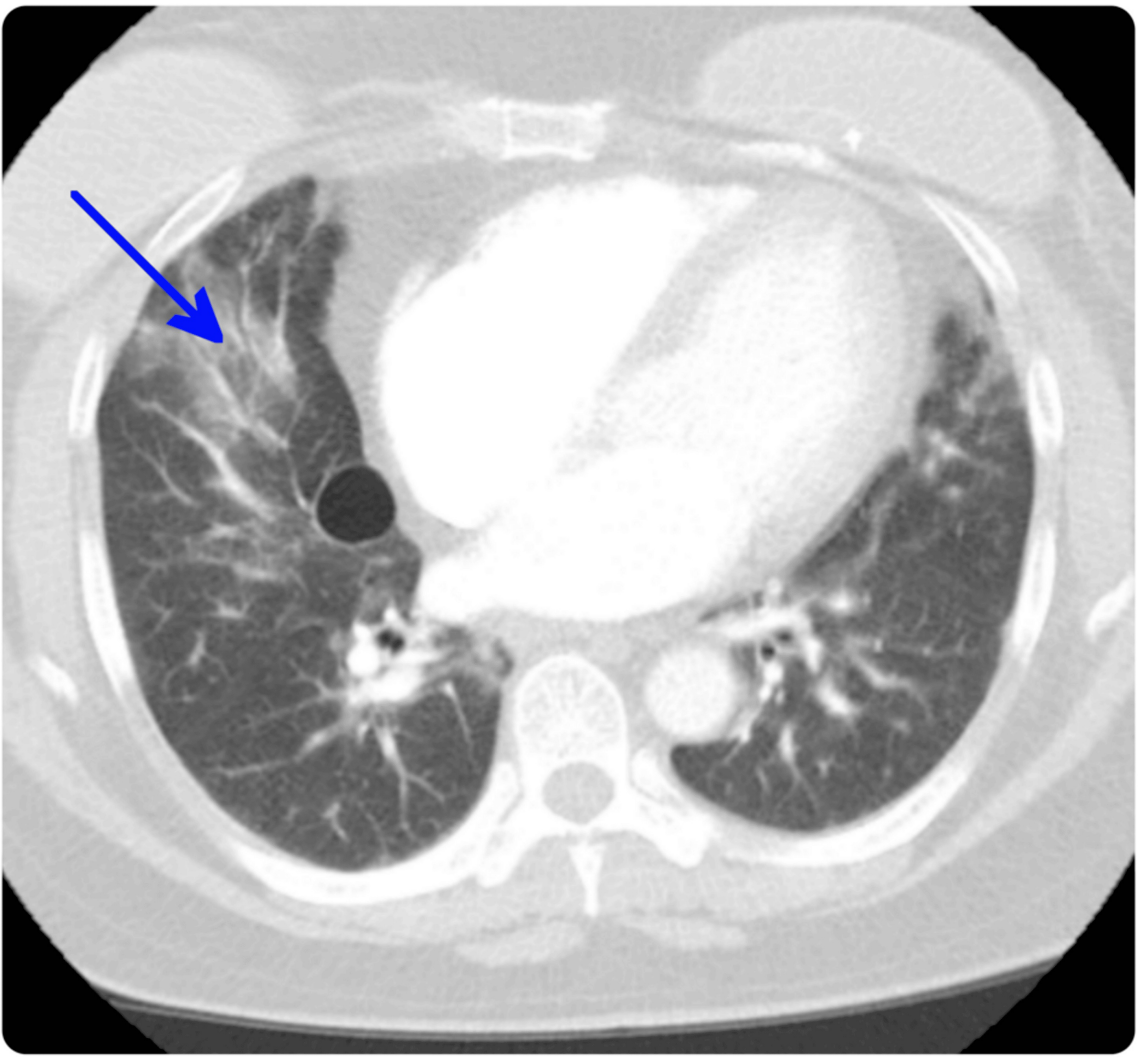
CT image from 2021 showing bilateral ground-glass infiltrates predominantly in the lower lobes, without honeycombing, consistent with active inflammatory interstitial lung disease (arrow).
